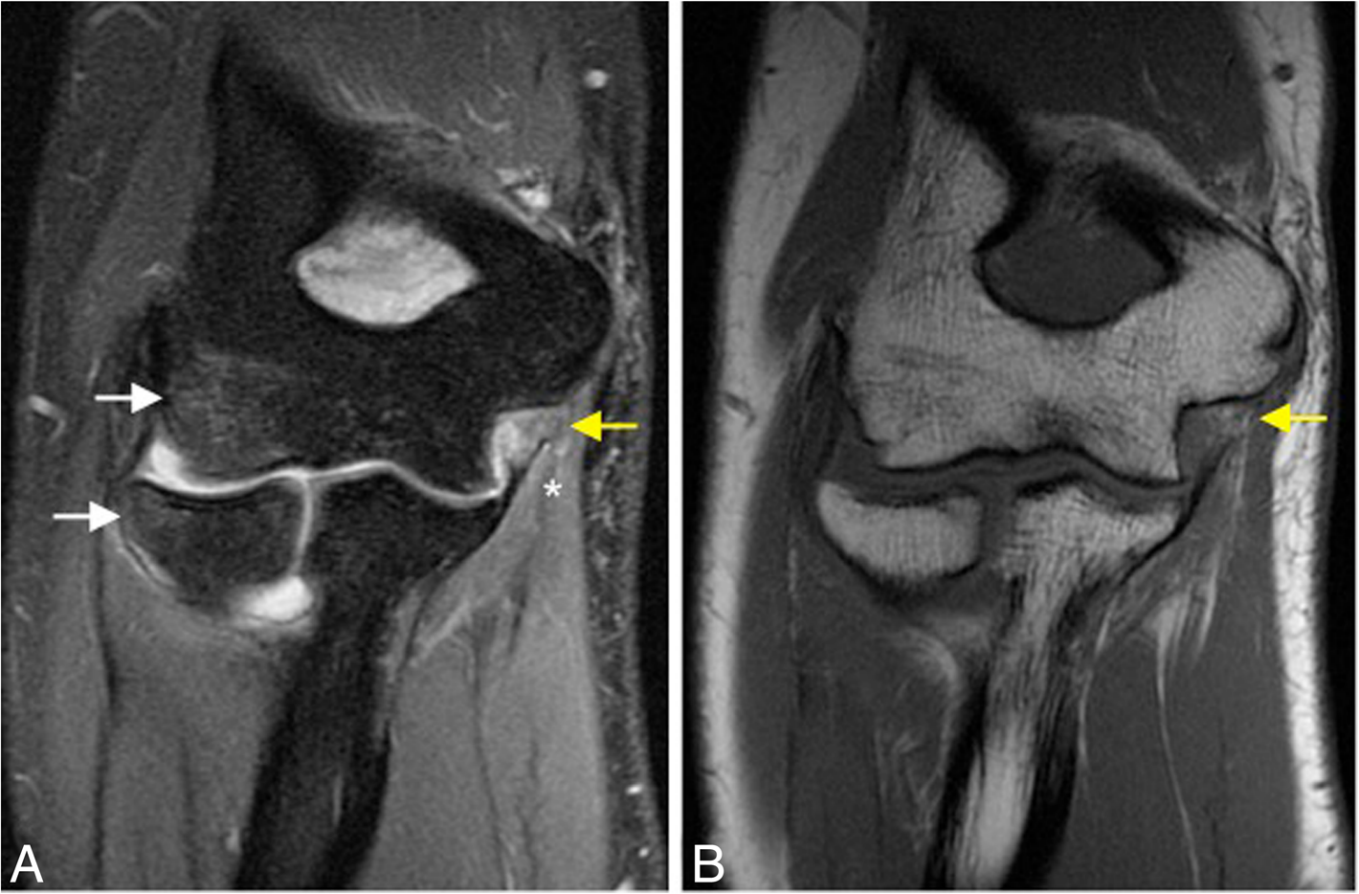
A 23-year-old man with pain and medial instability after a fall on the outstretched arm. Coronal FS PD-weighted MRI (a) and coronal T1-weighted MRI (b) showing an acute full-thickness proximal tear of the anterior bundle of the medial collateral ligament complex (yellow arrows), a flexor digitorum superficialis muscle edema (white asterisk), a radial head and posterior capitellar contusions (white arrows), and joint effusion
